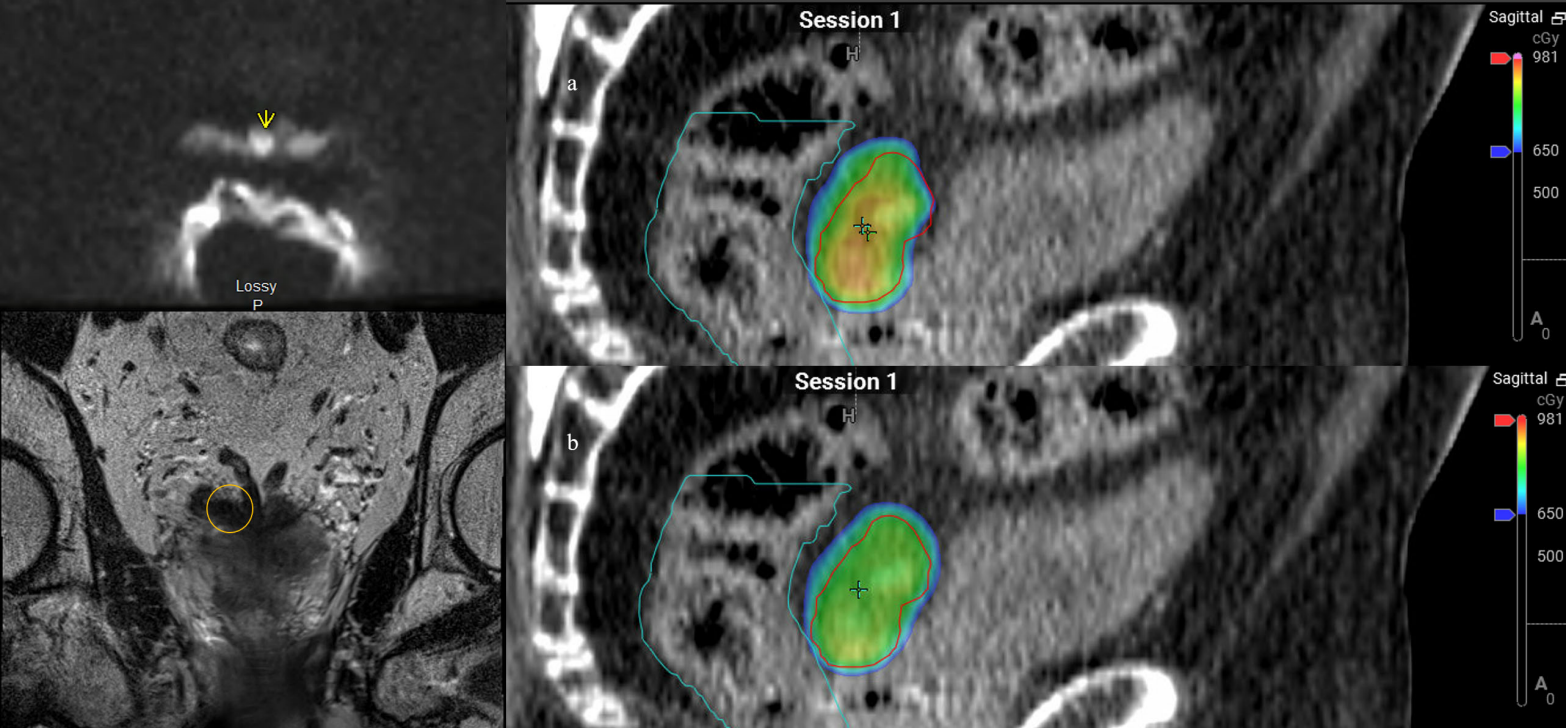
Patient 4. Left panel: diffusion weighted imaging with arrow indicating area of restricted diffusion and correlating location circled on coronal MRI. Right panel: Dose color wash of first fraction: (A) scheduled plan, (B) adapted (delivered) plan; red = PTV, cyan = rectum.
